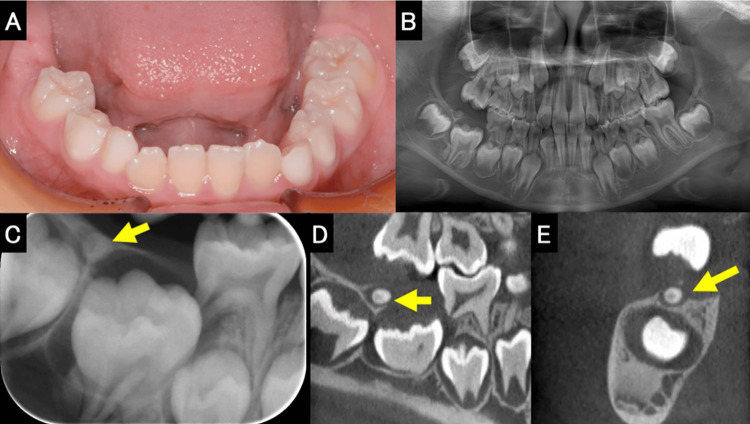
Intraoral photograph and radiographs (A) Intraoral photograph at first medical examination. The first molar on the right side of the mandible has not erupted. (B) Panoramic photograph. The roots of the first molars on both sides were complete. (C) Dental photograph at first medical examination. The yellow arrow indicates a spherical tooth-like hard tissue between the first and second molars on the right side of the mandible. (D, E) Dental CBCT images at first medical examination. The yellow arrow indicates a spherical tooth-like hard tissue. CBCT, cone beam computed tomography
